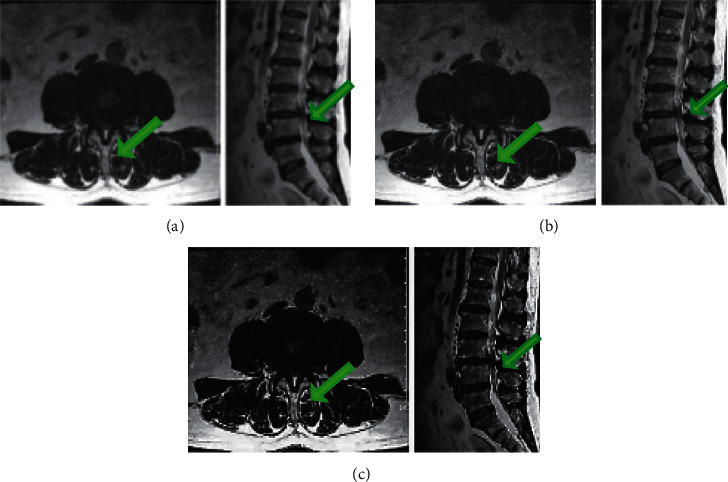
MRI images of the lumbar spine of LSS patients processed by different algorithms. (a–c) The conventional lumbar spine MRI image, the lumbar spine MRI image processed by the U-net algorithm, and the lumbar spine MRI image processed by the artificial intelligence-based U-net registration algorithm; the green arrow points to the lesion.
